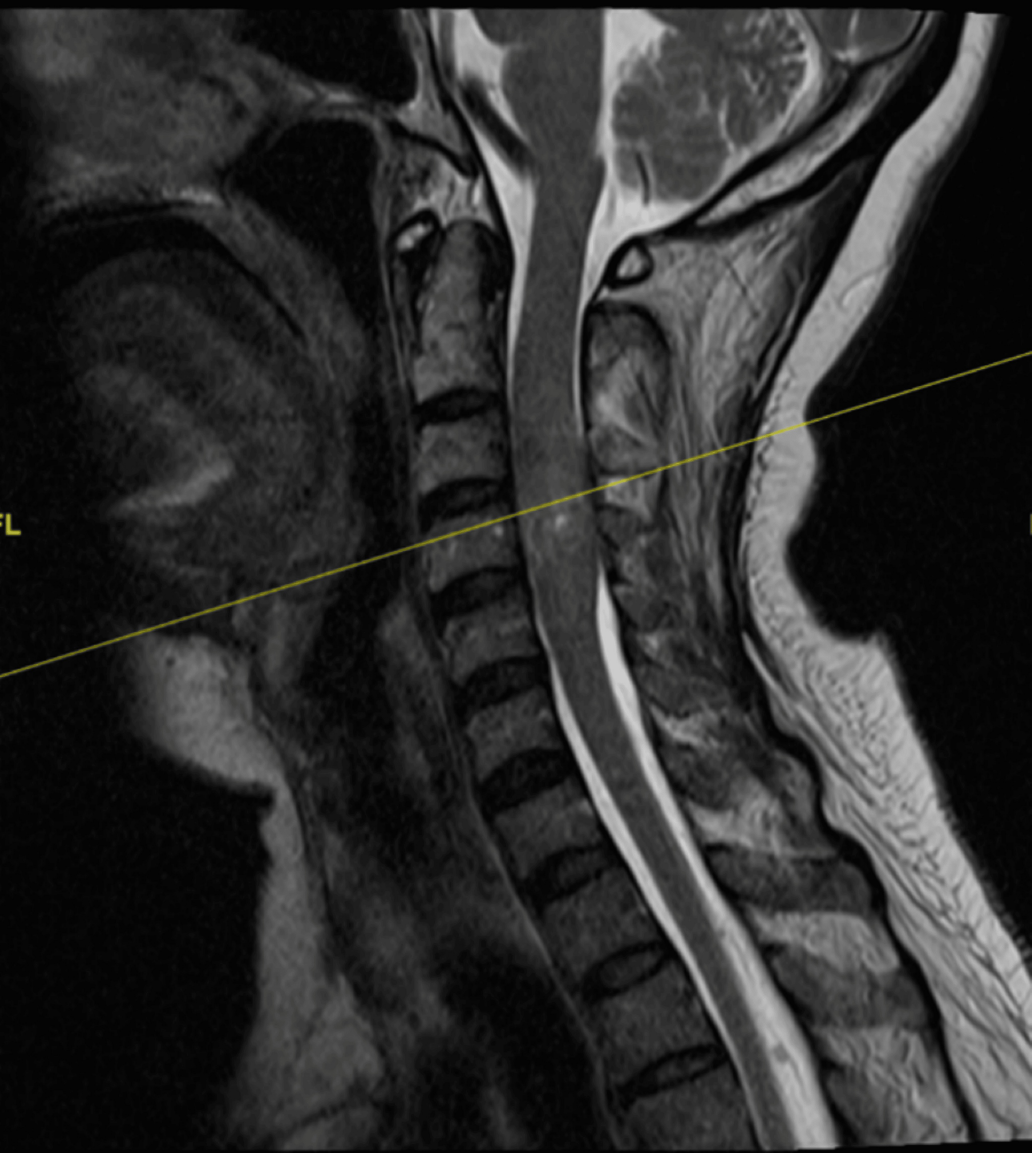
Pre-operative sagittal T2-weighted MRI of intradural extramedullary lesion at C3-4 level.
